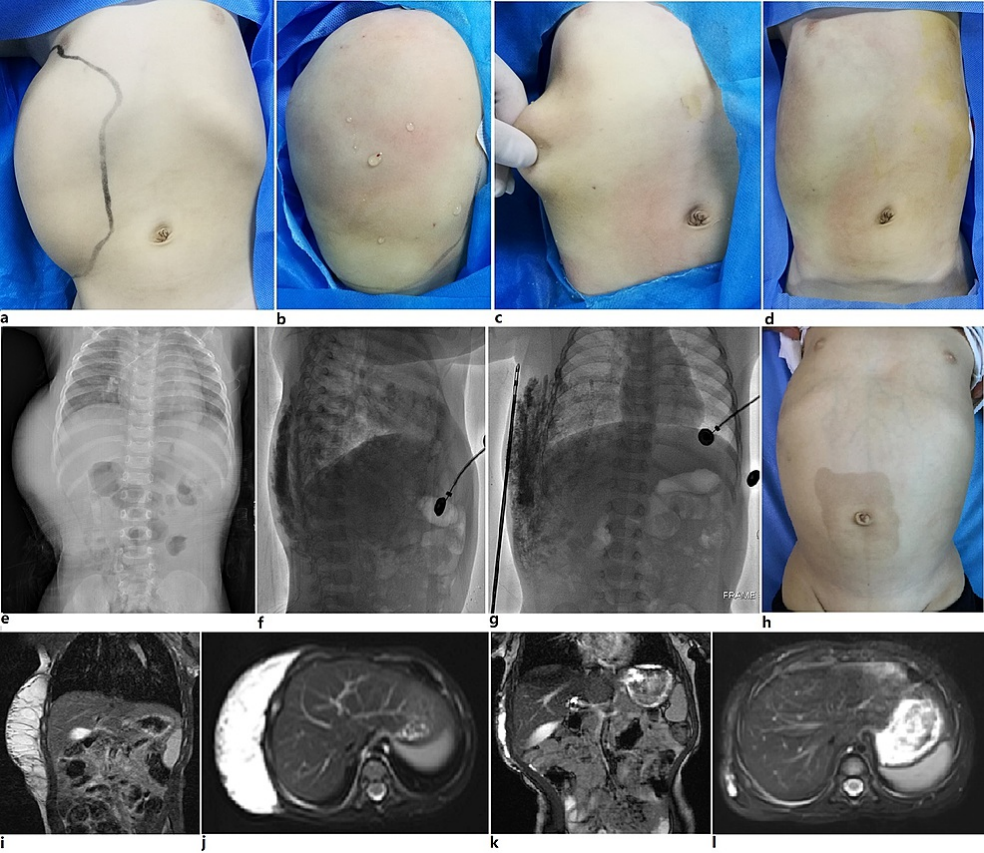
Mixed cystic lymphatic malformation. Details of the liposuction-like sclerotherapy on photographic and imaging view. Panel a, e, i, and j: An extensive mixed cystic lymphatic malformation of the right thoracic and abdominal wall. The position and extent of the lesion were topographically marked. Panel b: Showing the tumescent infiltrated lesion area. Panel c: During the vacuum-assisted liposuction-like operation, a pinch test was used to judge the endpoint. Panel d: At the endpoint of operation, the treatment area became smooth and flattened. Panel f and g: Showing the diffusion of the mixture of contrast medium and bleomycin in the treatment area, confirmed on lateral and frontal fluoroscopic imaging. A 2-mm in diameter, sharp, triple hole (Mercedes) cannulas in double row configurations were noticed on Panel g. Panel h: Showing the cosmetic appearance eight months after the operation. Panel k and l: Showing the MRI eight months after the operation. The result of treatment in this patient was graded as sub-complete response.
